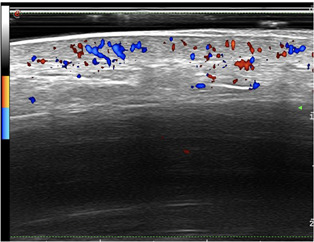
Case 3. Hypoechogenicity and increased Doppler flow in the dermis.
